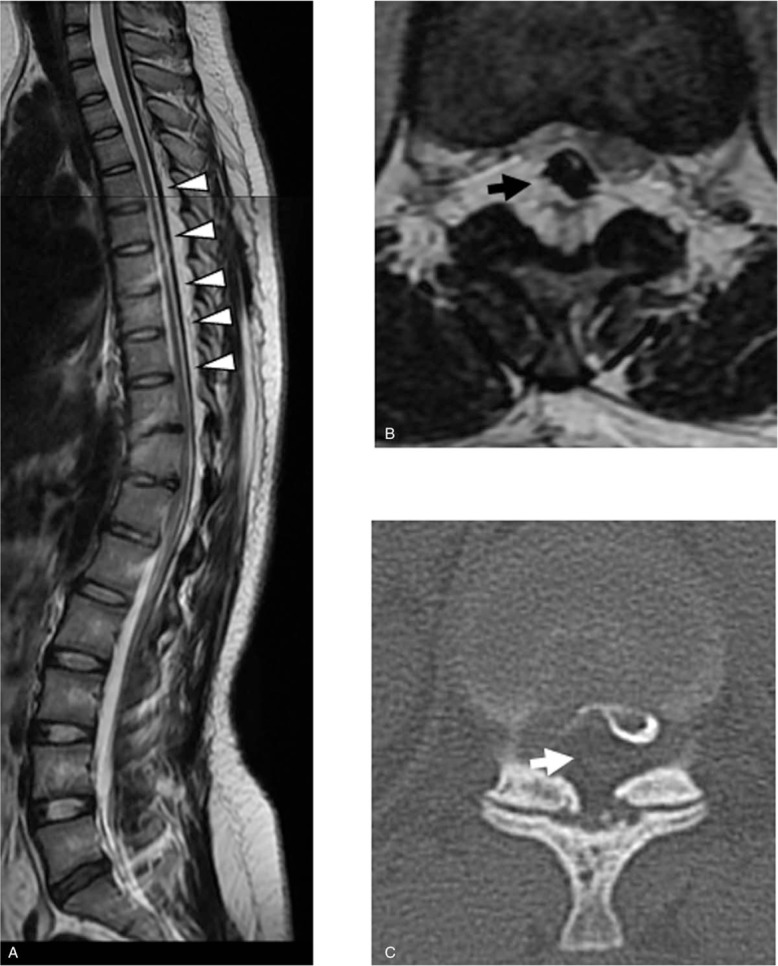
Preoperative magnetic resonance imaging. (A) Epidural ligament running from the cervical to lumbar spine observed on sagittal view (white arrowhead). (B) Fibrous band severely compressing the spinal cord (black arrow). (C) Thick fibrous ligament confirmed in the epidural space (white arrow).
